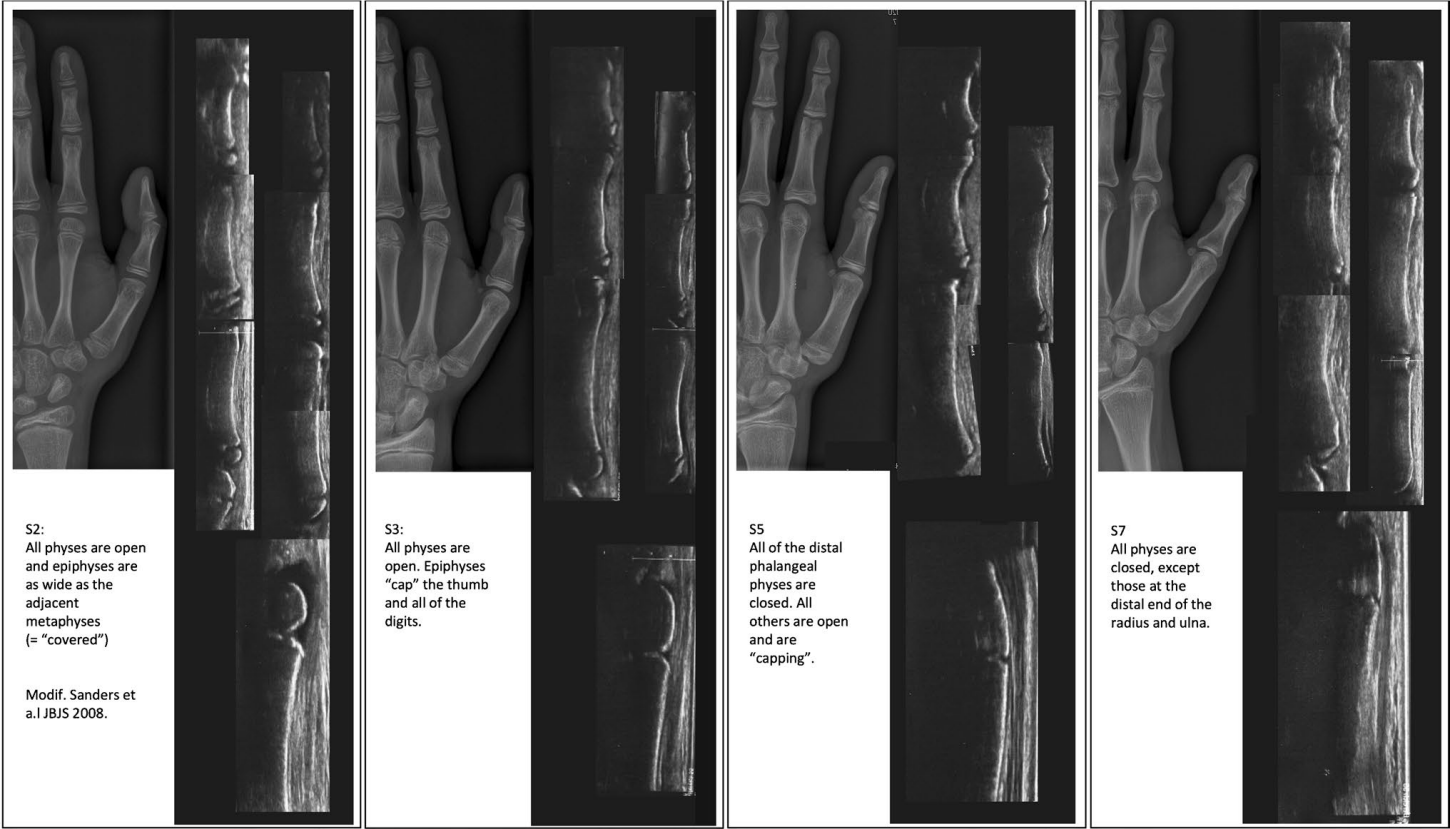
Examples for ultrasound performed of the thumb and index finger of the hand with Sanders stages 2, 3 5, and 7 with the corresponding X-rays of the hand
